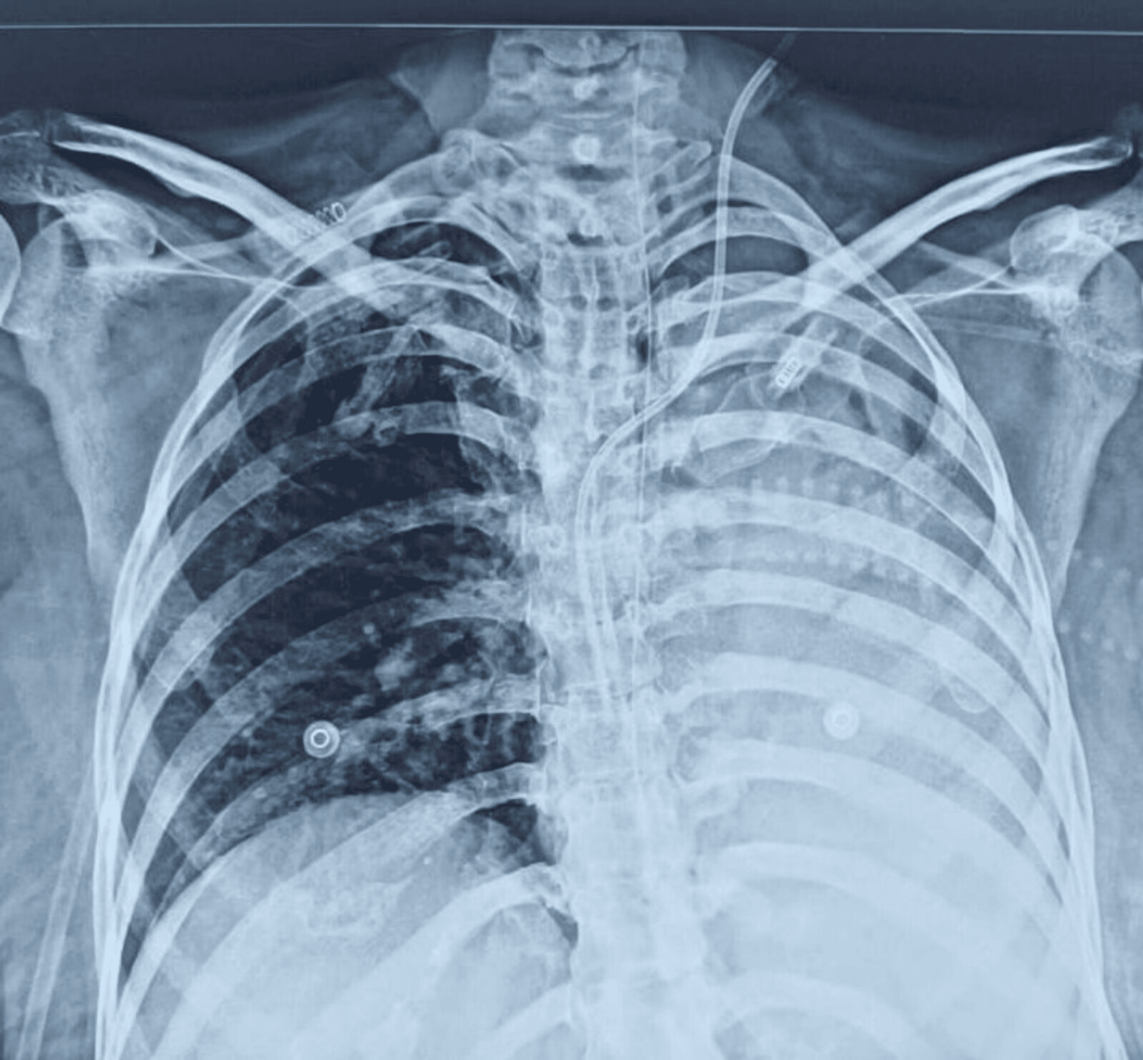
Chest radiograph taken at two weeks from transfer to our facility, where the patient had developed left-sided ventilator-acquired pneumonia. This resolved in five days.
